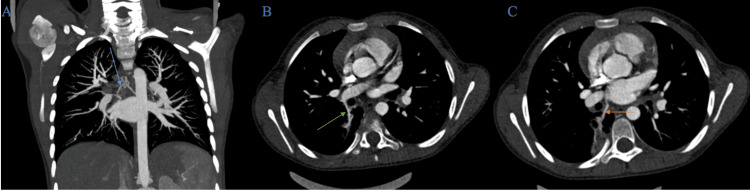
Chest CT angiography (mediastinal window) in coronal plane with maximum intensity projection (A) and axial plane (B, C) A systemic arterial feeder arising from the anterior aspect of the descending thoracic aorta (blue arrow), coursing toward the right lower lobe and entering the consolidation, is seen. A branch coming from the right inferior pulmonary vein (green arrow) and an anomalous venous drainage channel draining directly into the left atrium (orange arrow) is visualized.
